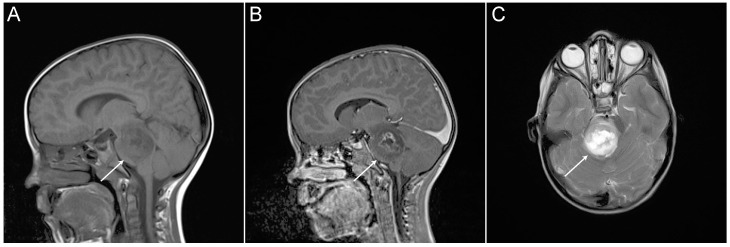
Magnetic resonance imaging (MRI) of DIPG tumor from an individual patient (white arrows). (A) Sagittal T1-weighted. (B) Sagittal T1-weighted. (C) Axial T2-weighted.
